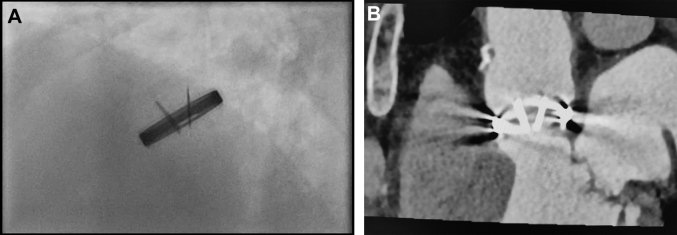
Fluoroscopic and CT Evaluation of the Implanted Bileaflet Mechanical Valve Fluoroscopic (A) and multidetector computed tomography (B) assessment of the implanted bileaflet mechanical valve. Both imaging modalities revealed reduced leaflet opening, with one leaflet at 60° to 65° and the other at 70° to 75°. According to the manufacturer, the leaflets open to 85° when fully functional, whereas normally functioning ATS valves in vivo open to approximately 70°,^10^ highlighting the subtle restriction of the first leaflet.
